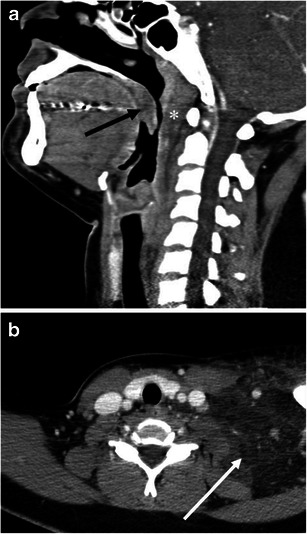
A 24-year-old female with systemic lupus erythaematosus and anti-phospholipid syndrome presenting with acquired angioedema. a The sagittal reformatted CE-CT shows diffuse retropharyngeal (*asterisk*) and soft palate oedema (*arrow*) causing upper airway encroachment at the level of the oropharynx. b The axial CE-CT shows extensive soft tissue oedema with fat stranding of the left lower neck (*arrow*)
